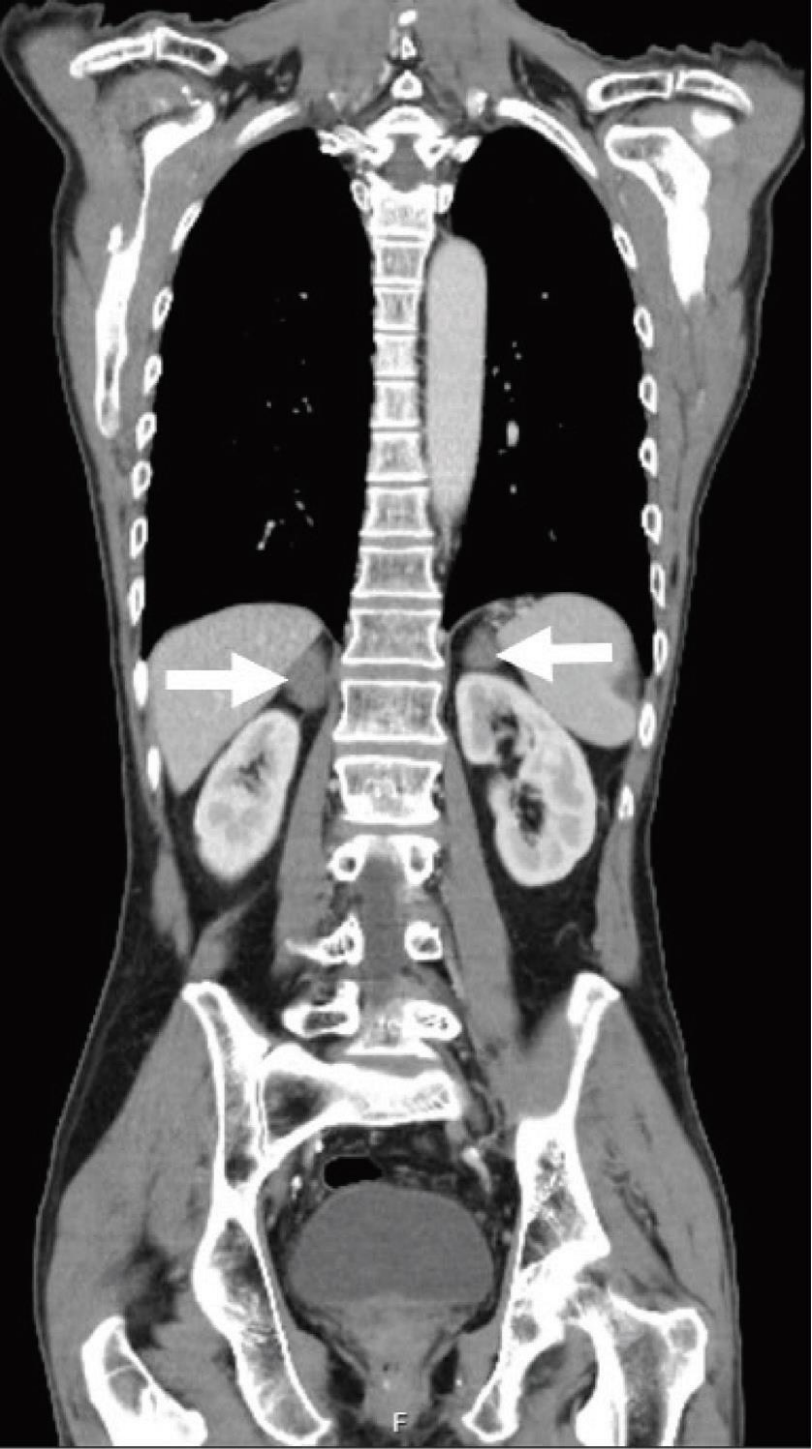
An abdominal computed tomography (CT) scan revealed bilateral adrenal enlargement (arrows) of unclear cause on initial imaging.
